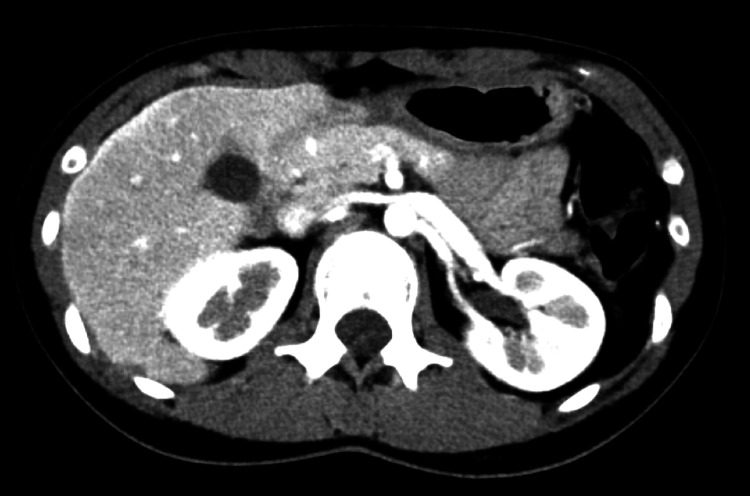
Early phase of contrast-enhanced CT of the abdomen. A contrast effect was observed along the liver capsule, mainly in the right lobe of the liver. CT: computed tomography.
